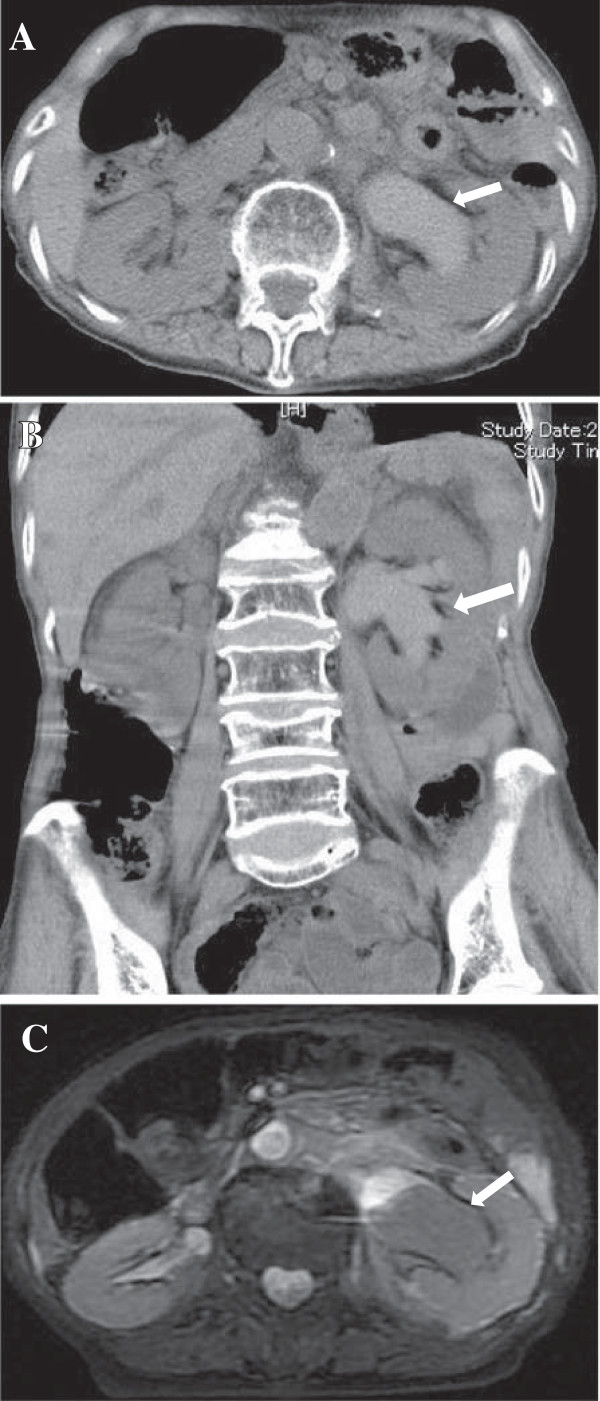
Plain abdominal computed tomography and magnetic resource imaging. Plain abdominal computed tomography revealed dilated left renal pelvis with retention of high-density contents (A: axial view, B: coronal view). T2-weighted abdominal magnetic resonance imaging revealed dilated left renal pelvis with retention of low-intensity fluid, which suggested the presence of blood (C).
